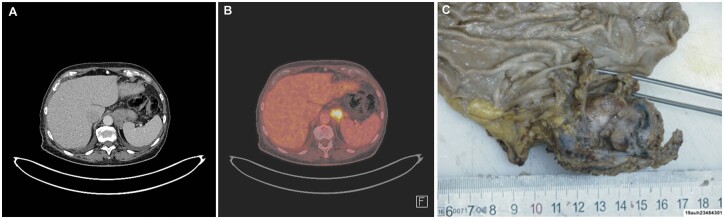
Computed tomography scan (A) and positron emission tomography-computed tomography scan (B) showed a 5-cm tumour located in close relation to the fundus of the stomach. (C) Photo of the resected material in Case 2.
